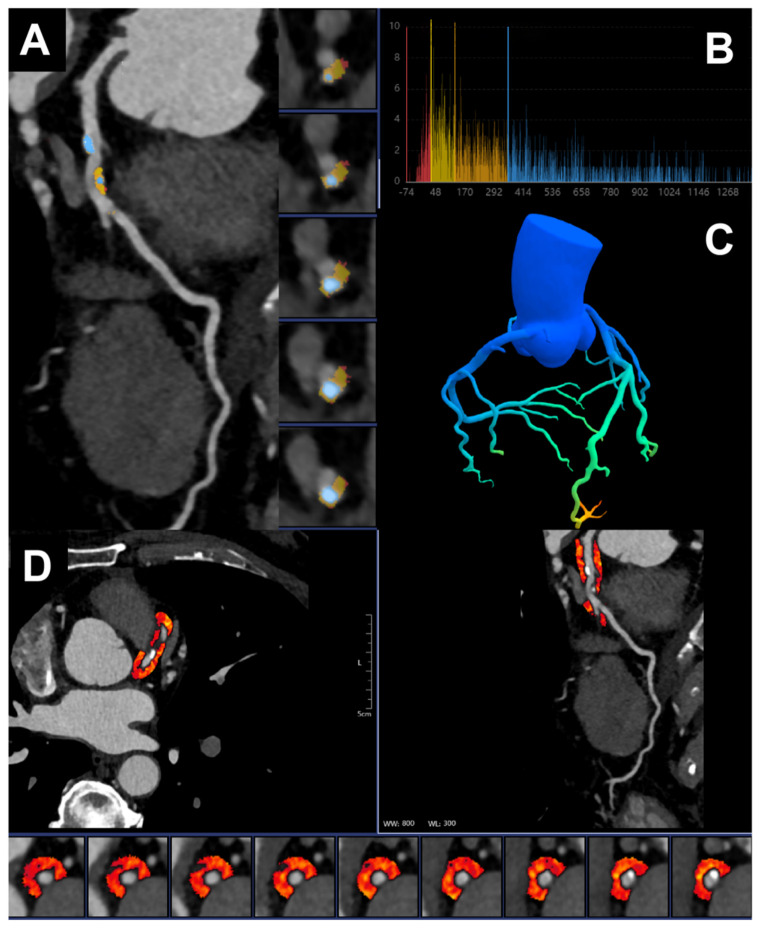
Representative multiparametric CCTA findings in a patient with DM. Curved planar reconstruction demonstrated mixed plaque in the proximal left anterior descending artery (A). Quantitative plaque analysis (B). CT-FFR value of 0.86 (C). Perivascular FAI of −69.2 HU (D). This patient experienced MACE during follow-up.
